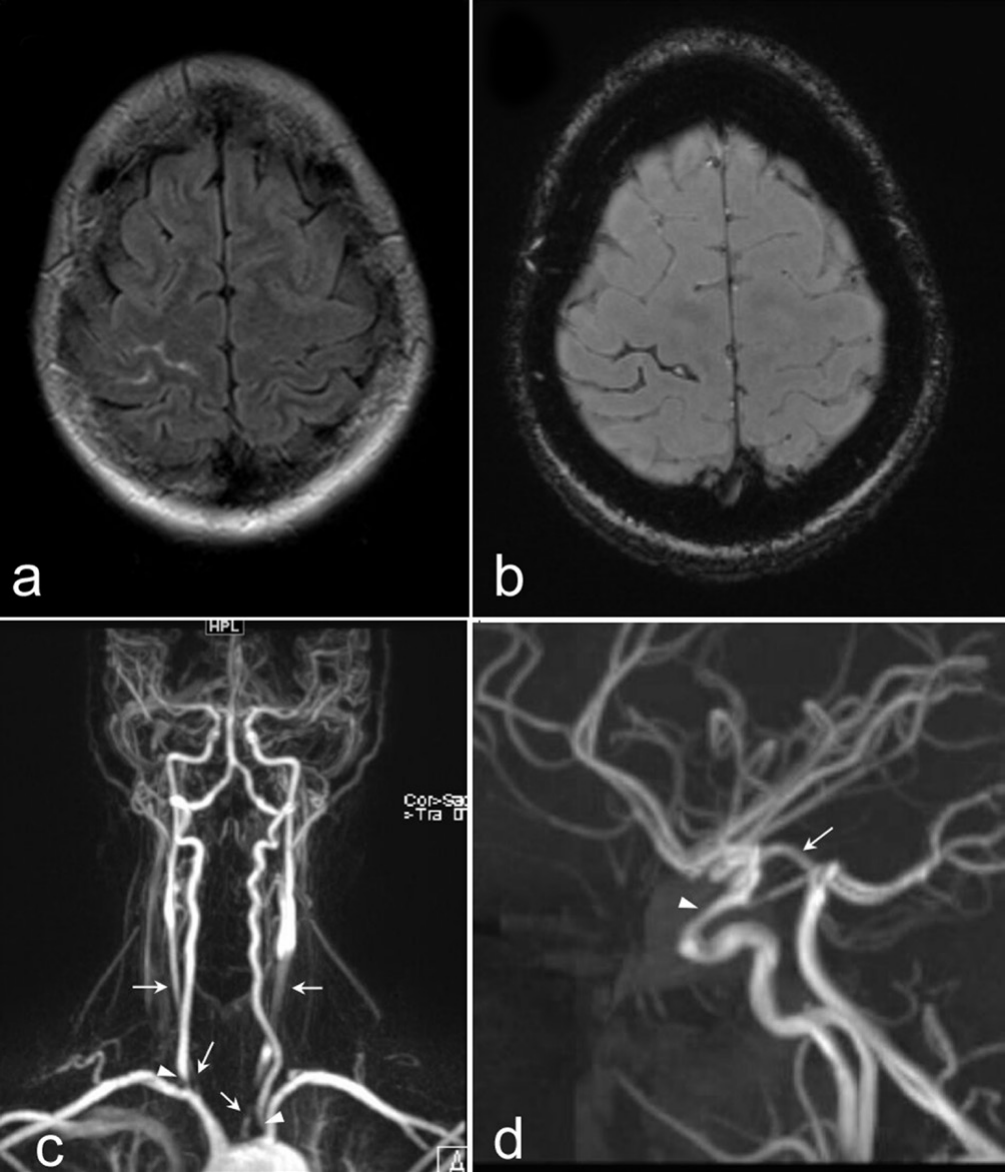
(a) Axial T2 FLAIR MRI of the brain and (b) corresponding SWI demonstrating small volume right frontal lobe subarachnoid hemorrhage (c) Coronal MIP of MRA of the head and neck showing severe stenoses of the bilateral common carotid arteries (arrows) and at the origins of the vertebral arteries (arrowheads) (d) Sagittal MIP of brain MRA demonstrates narrowing of the cavernous and supraclinoid segments (arrowhead) of the right internal carotid artery with prominent right posterior communicating artery (arrow), which may explain altered vascular supply and chronic frontotemporal hyperperfusion. No aneurysm was identified. FLAIR, fluid-attenuated inversion-recovery; MIP, maximum intensity projection; SWI, susceptibility weighted imaging.
